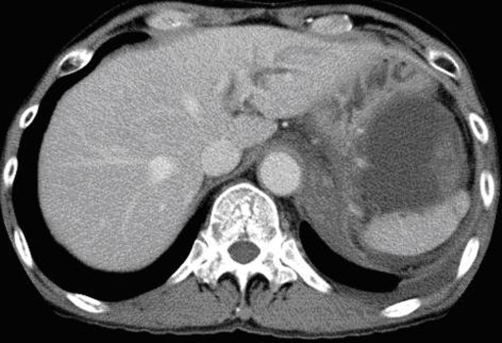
CT scan demonstrated high density structure in the cyst.
